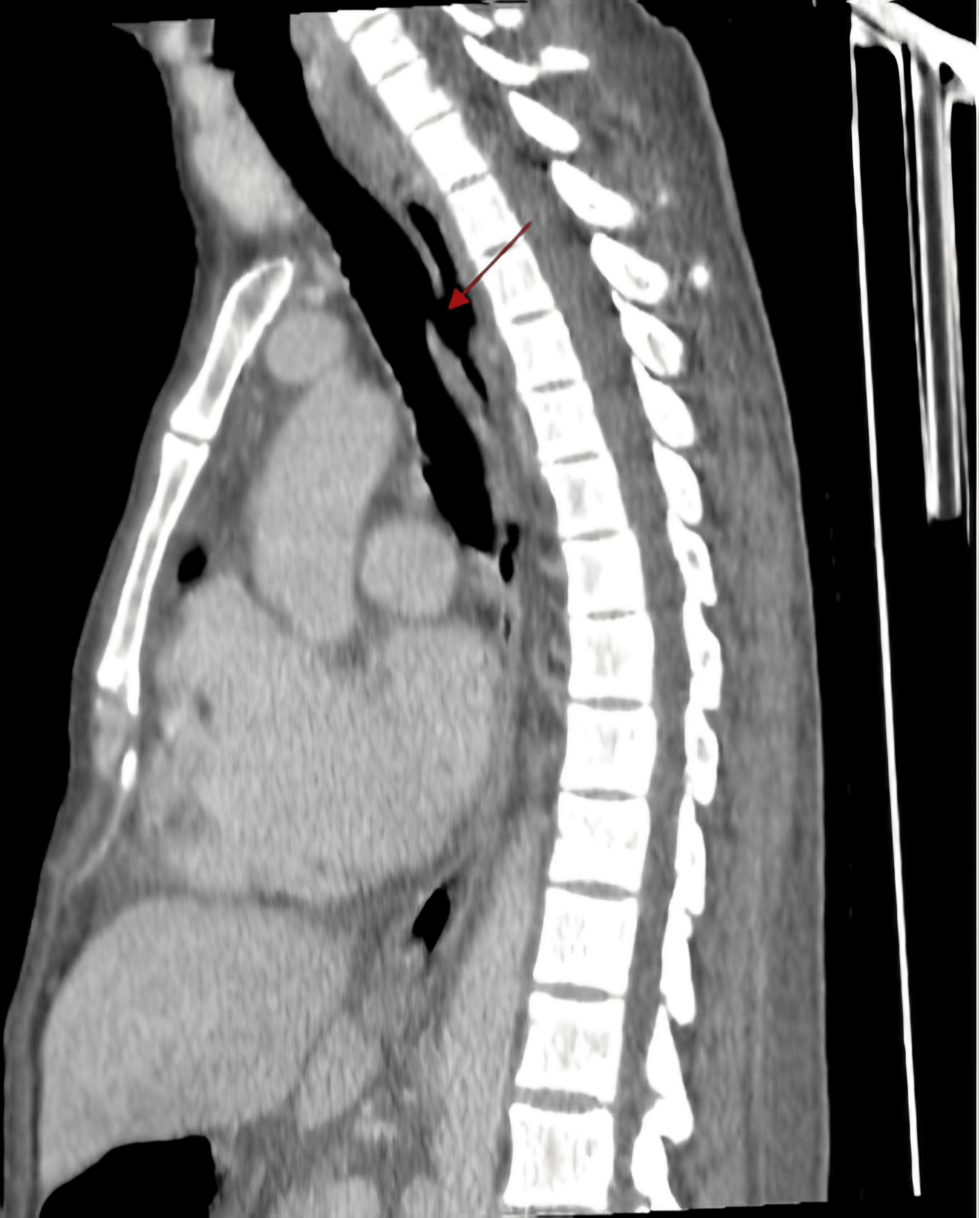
Sagittal CT scan image with contrast showing the H-type trachea esophageal fistula (red arrow).
